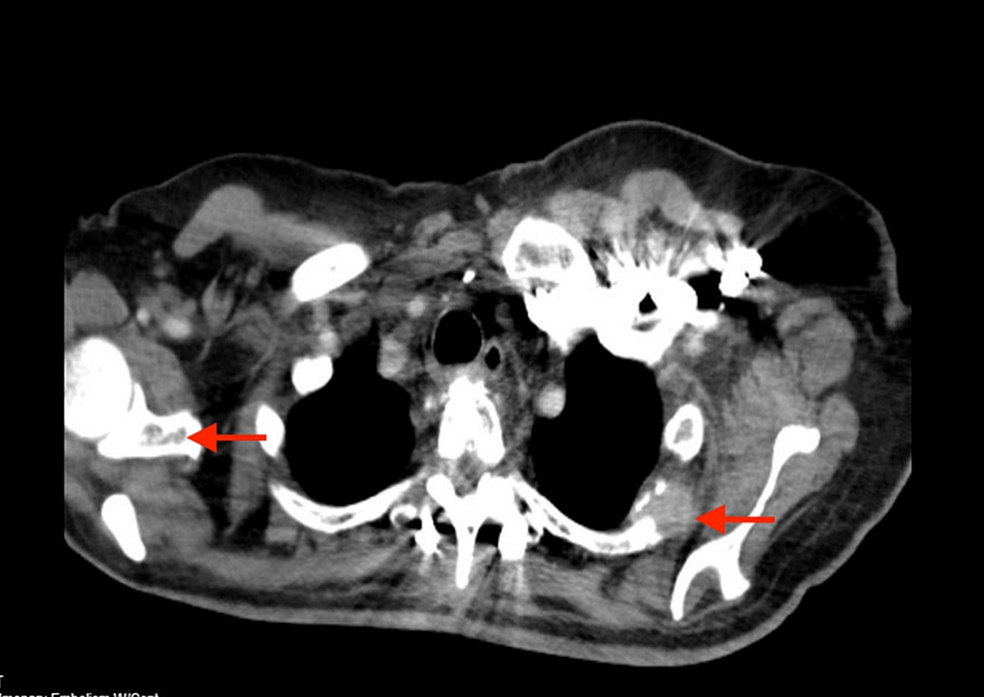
Computed tomography - pulmonary embolism of the thorax with contrast showing intraosseous metastasis (arrows) involving the left second rib and glenoid of the right scapula
